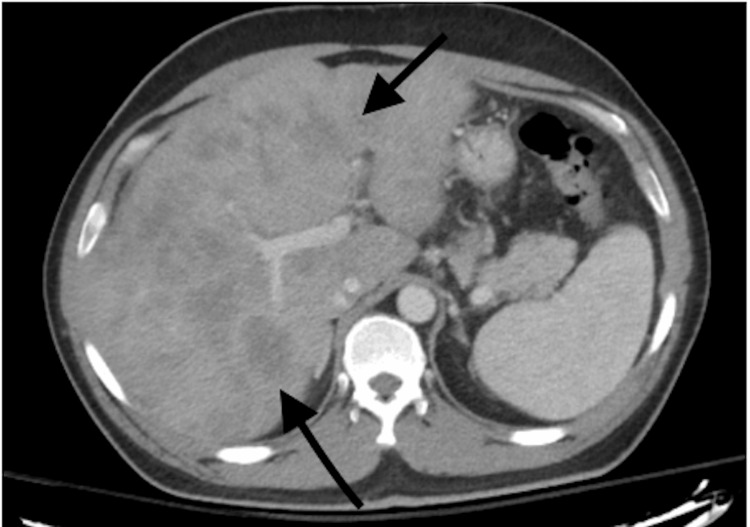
CT abdomen and pelvis with contrast, axial view, demonstrating diffuse liver metastases.
